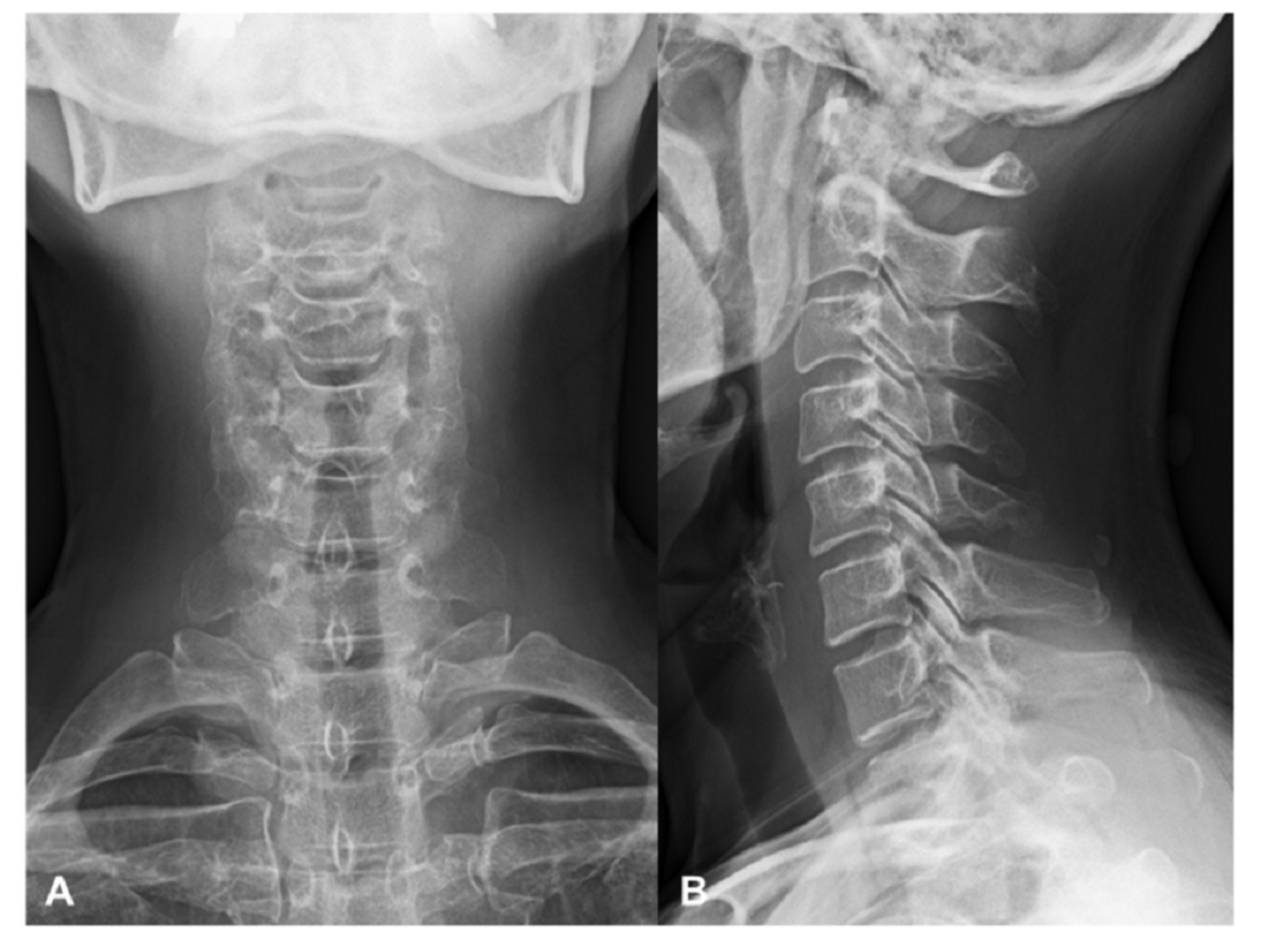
Plain radiographs of the cervical spine on initial presentation Anteroposterior (A) and lateral (B) radiographs showing no apparent abnormality
